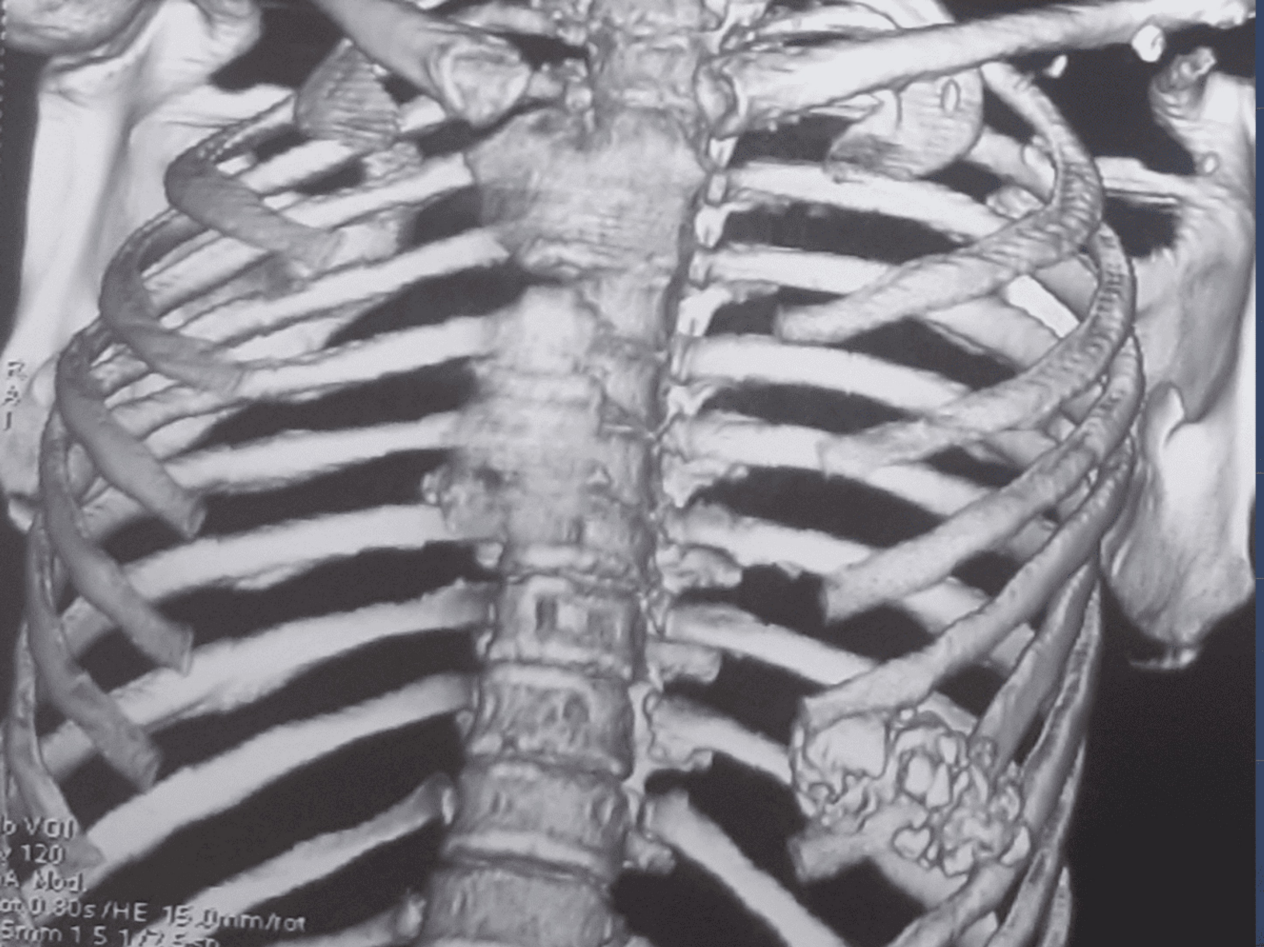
CT imaging of the chest wall showing the costal osteochondroma with no invasion of the pleura Three-dimensional reconstructed CT scan of the chest showing a costal osteochondroma arising from the left sixth rib. The lesion extends toward the intercostal spaces without evidence of pleural, pulmonary, or mediastinal invasion.
